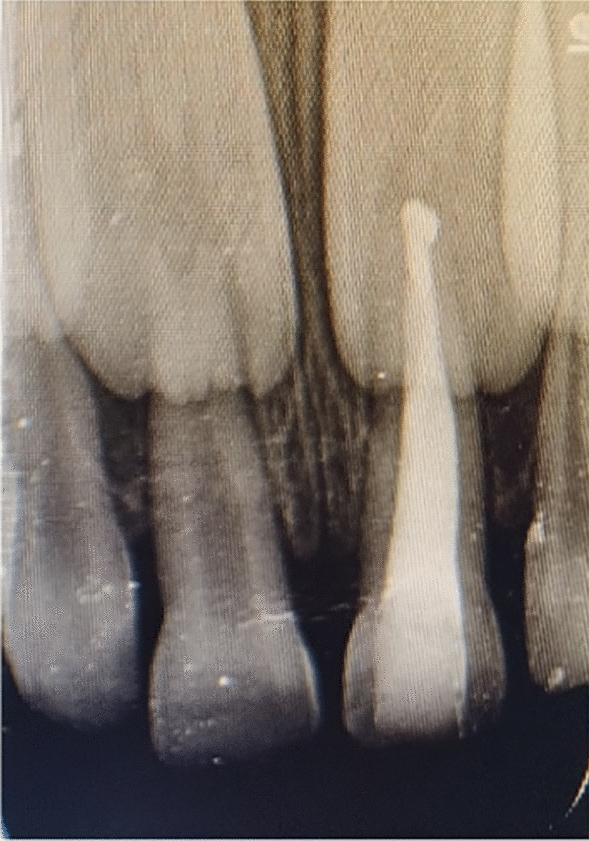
Postoperative radiograph of tooth 61
